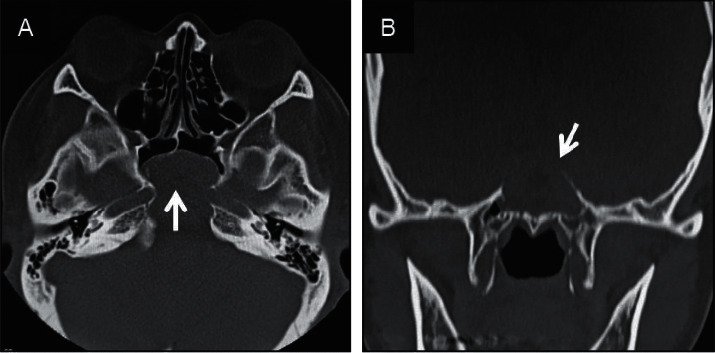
Noncontrast computed tomography imaging of the paranasal cavity at the initial diagnosis. (a) Axial and (b) coronal views. We confirmed the presence of soft tissue contrast, suggesting a retention cyst at the rear sphenoid sinus, with thinning at the sella turcica and left internal carotid artery and elimination of the clivus (white arrows).
